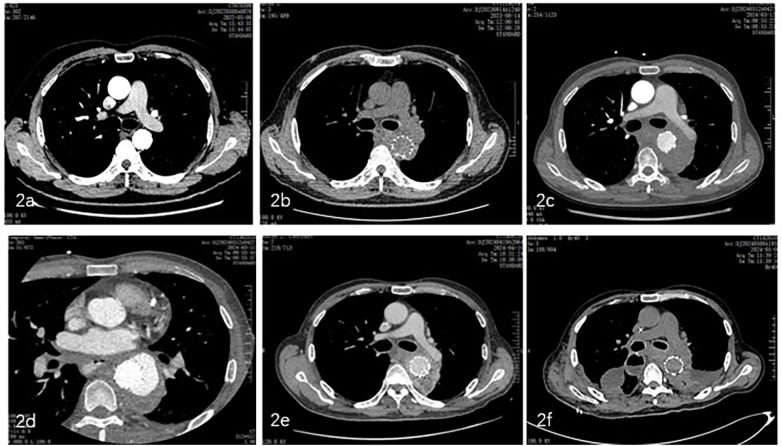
(a) chest CT scan six months after TEVAR. (b) Two years after TEVAR, fluid accumulation around the stent graft; (c,d) Two and a half years after TEVAR, the patient was found to have increased fluid accumulation accompanied by contrast extravasation on a CT scan during a follow-up for hematemesis. (e) Follow-up aortic CTA after re-implantation of a covered stent shows persistent fluid around the stent. (f) Chest CT scan for postoperative hemoptysis after undergoing gastric substitution for esophagus via the right thoracic cavity due to hematemesis.
